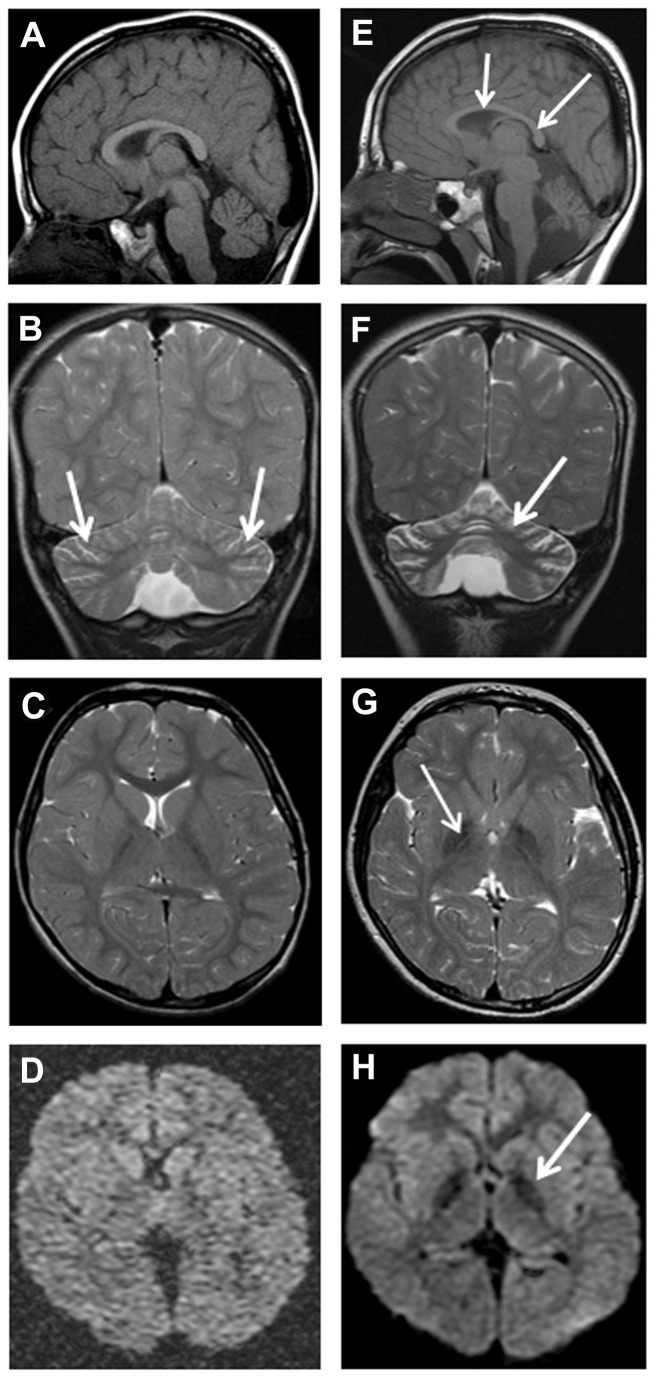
Patient F5 (P1) MRI, age 4 years 2 months (A-D), and 7 years 6 months (E-H). Sagittal T1-weighted (A and E), coronal T2-weighted (B and F), axial T2-weighted (C and G) and axial diffusion (D and H) sequences showing mild cerebellar cortical atrophy with mildly prominent folia (arrows in C). There is also simple corpus callosum (arrows in E). (F) Coronal T2-weighted reveals progressive cerebellar cortical atrophy and gliosis, with widening of folia and increased signal in the residual cerebellar cortex (arrow). Axial T2-weighted (G), and axial diffusion (H) sequences highlight iron deposition as reduction in signal intensity in the globus pallidi (arrows).
